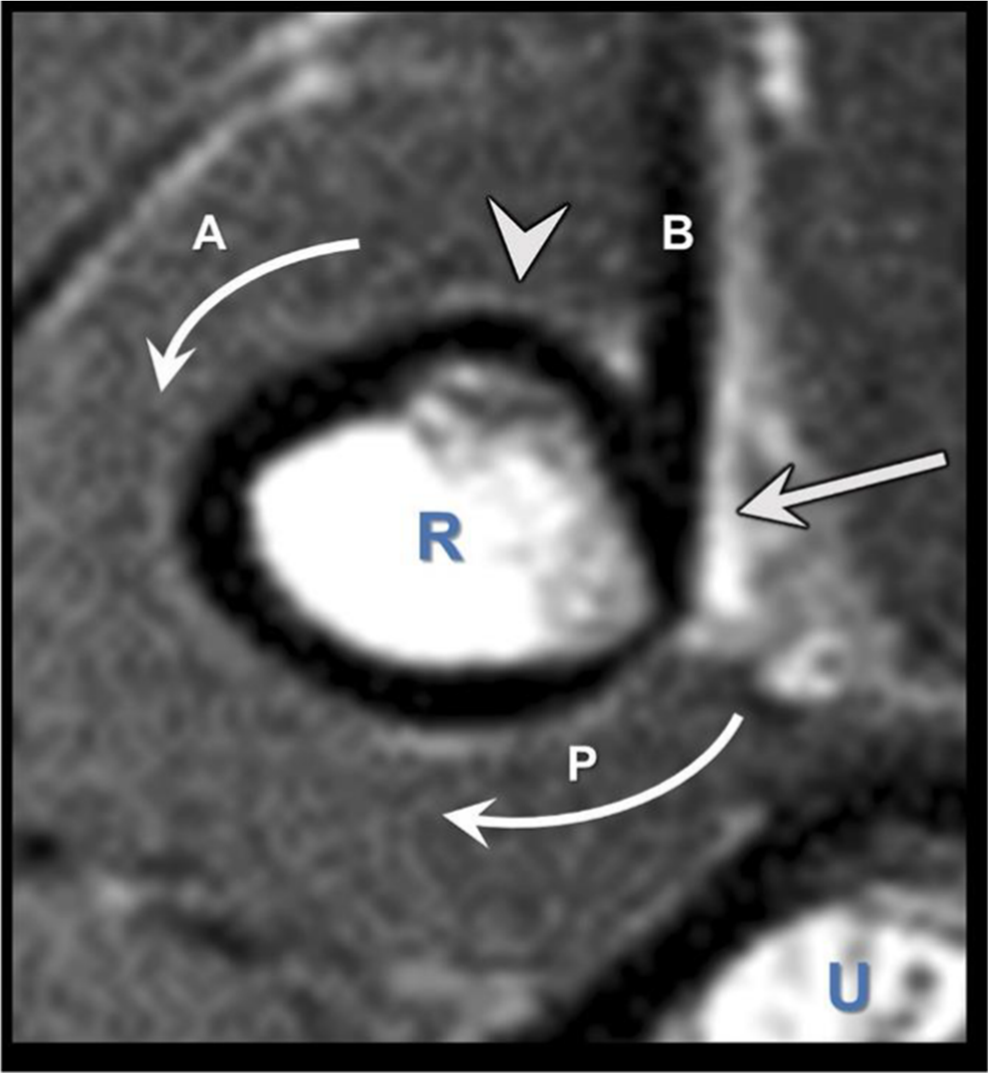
The magnetic resonance imaging (MRI) axial view through the insertion of the distal biceps. The arrow shows the biceps tendon (B). The anterior (A) and posterior (P) curved arrows show the arc of the radial tuberosity. The protuberance (arrowhead) of the radial tuberosity is found anterior to the tendon insertion and is thought to function as a mechanical cam in increasing the supination moment of the biceps. R, radius; U, ulna. (Reprinted from *J Bone and Joint Surgery*, 2015, doi.org/10.2106/JBJS.N.01221, Schmidt CC, Brown BT, Williams BG, Rubright JH, Schmidt DL, Pic AC, Nakashian MR, Schimoler PJ, Miller MC with permission from Wolters Kluwer (The Importance of Preserving the Radial Tuberosity During Di... : JBJS (lww.com))
